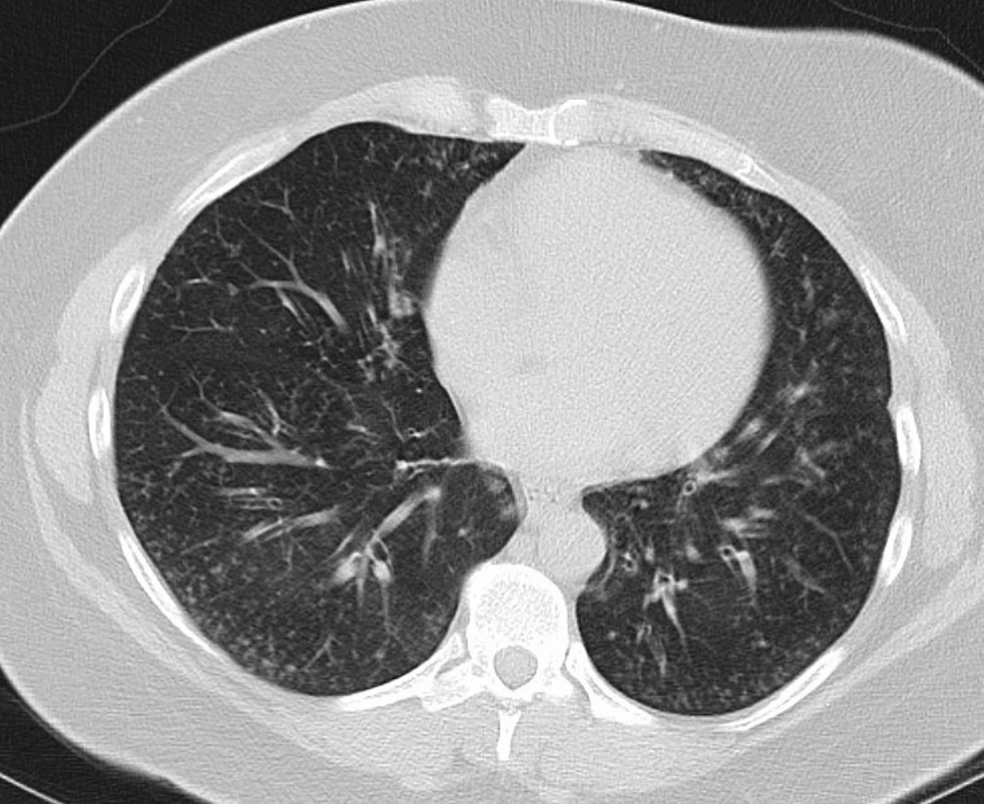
Axial CT image showing multiple bilateral centrilobular and tree-in-bud nodules, concerning for small airway disease.
